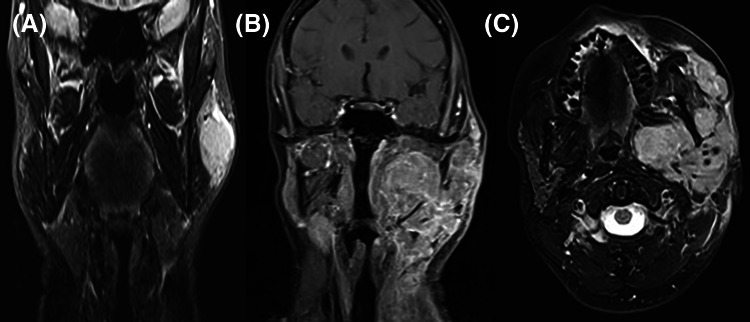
T2-weighted MRI images from left to right: a Pre-operative staging image in the coronal plane demonstrates a well-defined tumour in the left parotid gland. b, c Imaging one month later demonstrates extensive tumour invading into the lower neck, mandible and skull base in both coronal and axial planes
